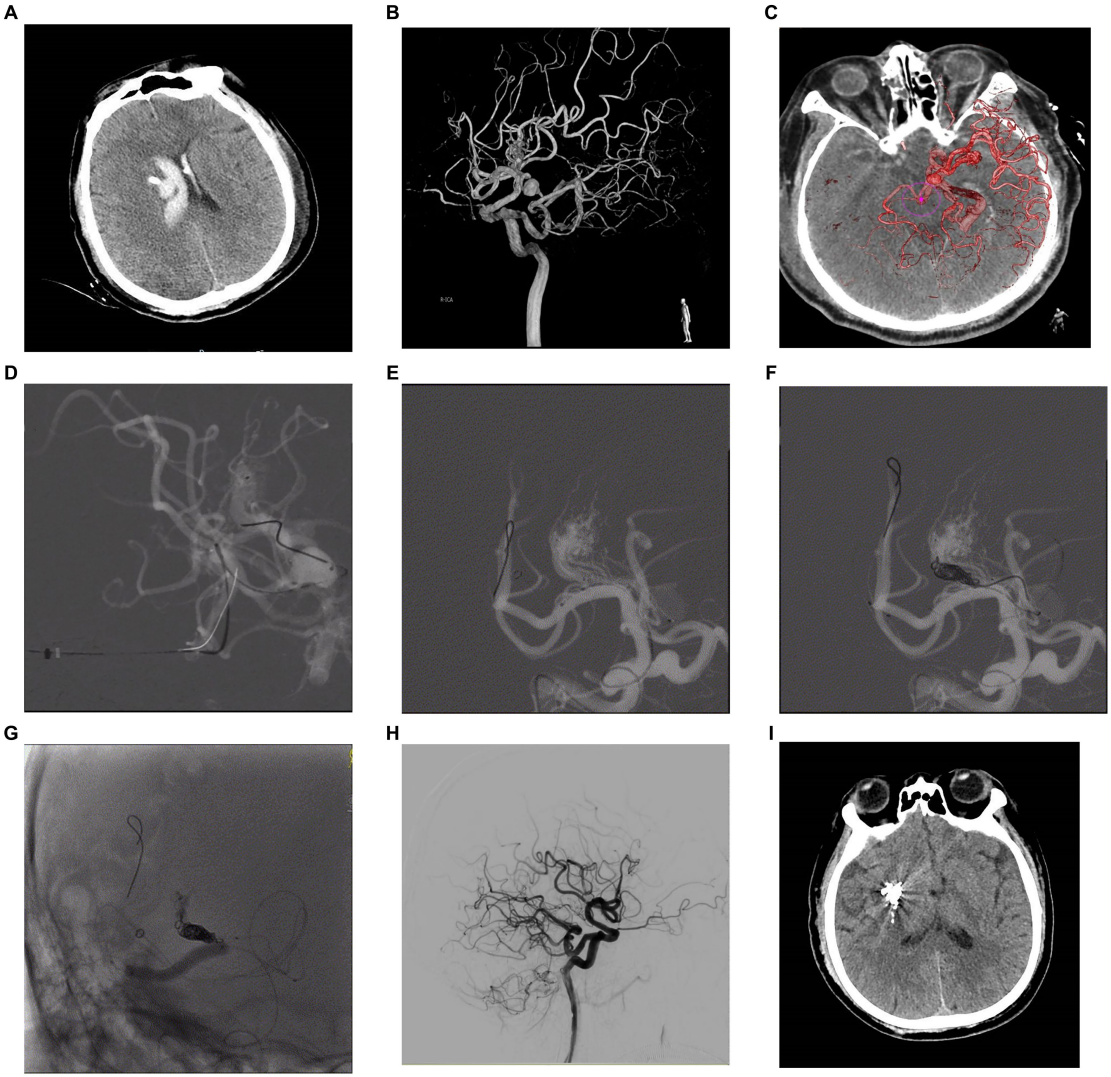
A 57-year-old male patient presented with sudden headache and acute confusion of consciousness, who was performed emergency external ventricular drainage, and, 4 weeks later, the AVM was embolized by using the TVA. Nonenhanced CT scan in axial view (A) showed acute hematoma in right thalamus with ventricular extravasation. Three-dimensional DSA (B) revealed an AVM supplied by anterior choroidal arteries and lenticulostriate arteries with a nidus measuring 1.0 cm. Venous aneurysm is presented in the venous drainage network of AVM. The deep drainage was conducted through the superior petrosus sinuses. The fusion image of three-dimensional DSA and Xper-CT (C) better delineated the location relationship of AVM nidus. Intraoperative road-map image in lateral view exhibits a 6F SOFIA intermediate catheter (MicroVention, Tustin, California) placed at superior petrosus sinuses and a microcatheter Apollo (EV3, Irvine, California) navigated as close as possible to the initial part of primary draining vein (D), then another microcatheter Headway Duo (MicroVention, Tustin, California) was placed to near the Apollo. Its tip was positioned between the most distal marker and the detachment zone of the Apollo. A ScepterC balloon (E) was placed in the right middle cerebral artery in preparation for blocking the blood supply to the perforator artery. Then, coils were placed in the draining vein through the Headway Duo microcatheter to create a plug (F). Intraoperative nonsubtracted single shot in AP (G) view showing the cast of Onyx (EV3, Irvine, California). After treatment, control DSA of the right ICA in lateral (H) view confirmed anatomic resolution of the lesion. Postoperative head CT (I) confirmed no intracranial hemorrhage and edema. AP, anteroposterior; AVM, arteriovenous malformation; CT, computed tomography; DSA, digital subtraction angiogram; ICA, internal carotid artery; TVA, transvenous approach.
